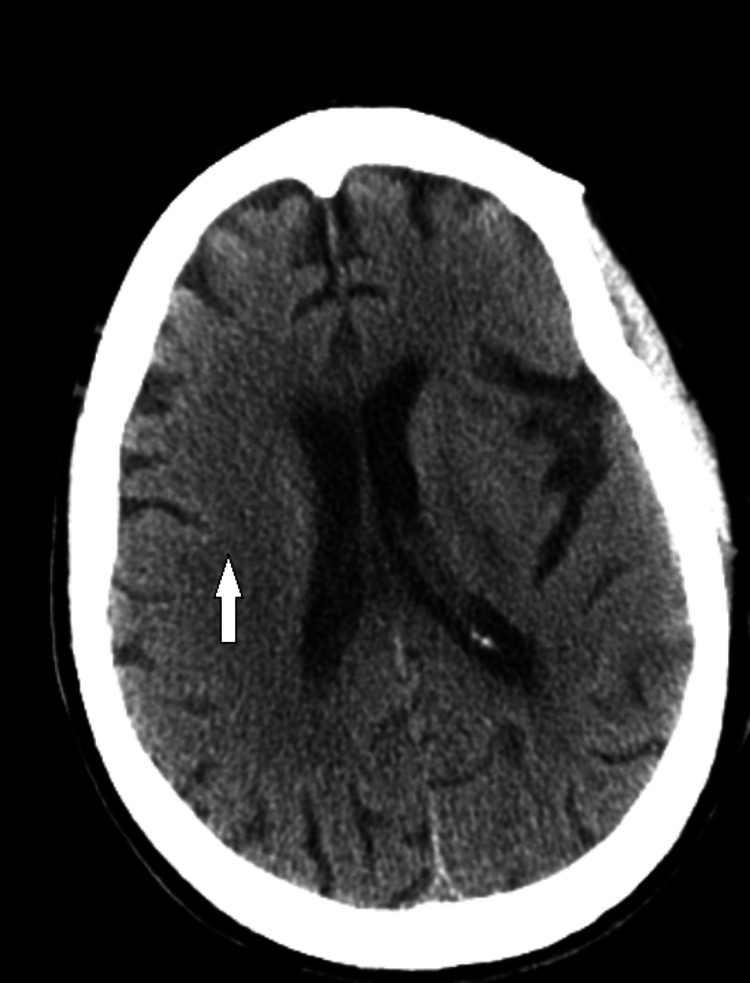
Computed tomography (CT) scan of the head showed moderate diffuse chronic senescent changes (white arrow)
